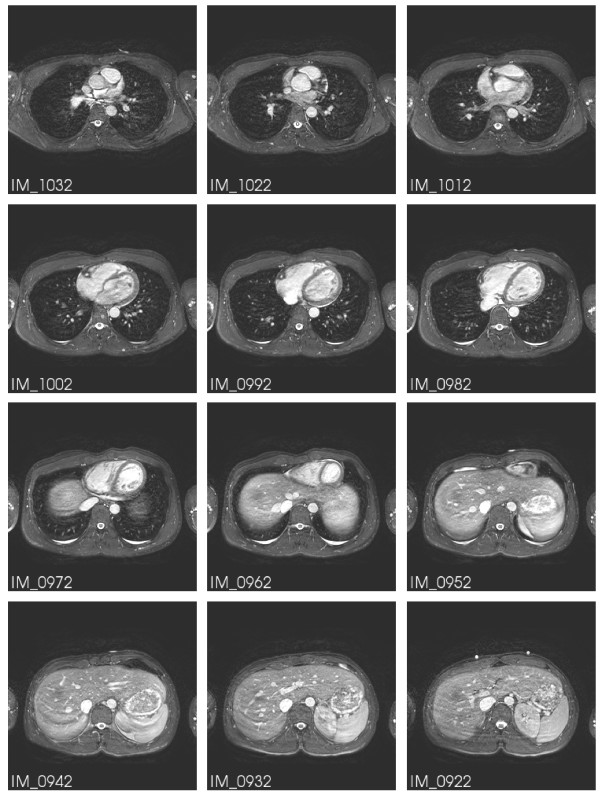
MR image stack of the heart at diastole. A subset of series 701. In IM_0922 the same markers are seen as in IM_0108 of Figure 1.
